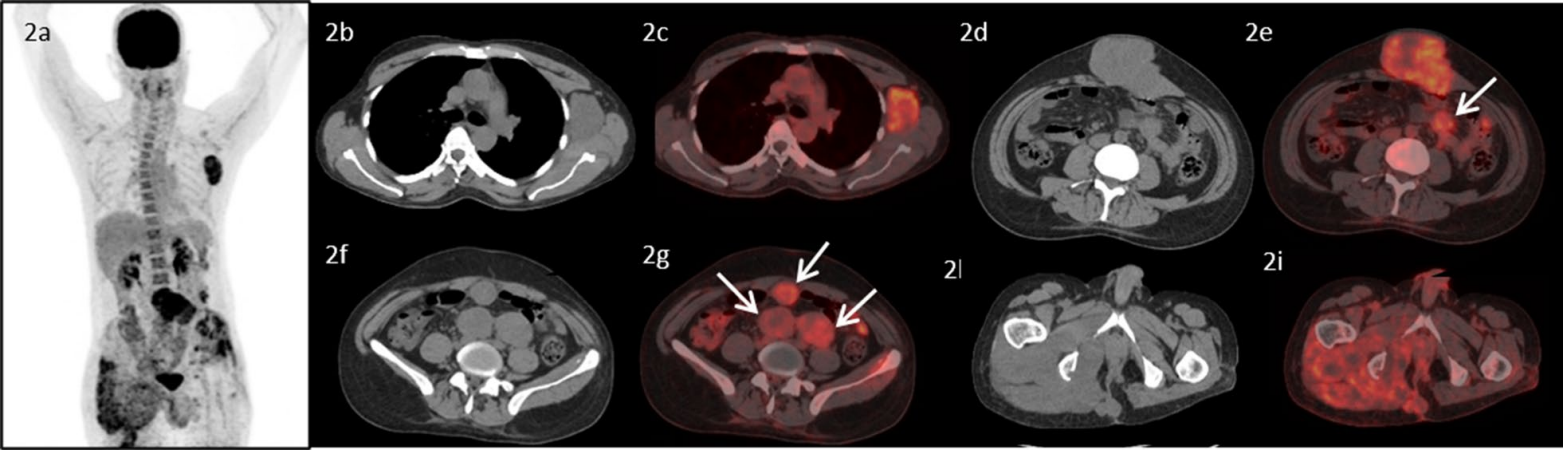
18F-FDG PET/CT images of the patient three months after initiation of therapy with imatinib. MIP image (2a) shows focal increased FDG uptake in the left axillary region, lower abdominal and pelvic regions, which increased since the previous PET/CT image (1a). Transaxial CT and fused PET/CT images show increase in size and metabolic activity of the of the left axillary lymph node (2b and 2c), anterior abdominal wall lesion (2d and 2e), with interval appearance of new omental/mesenteric deposits (2e, 2g, arrows). No significant interval change was noted in the lesion near the right ischium
